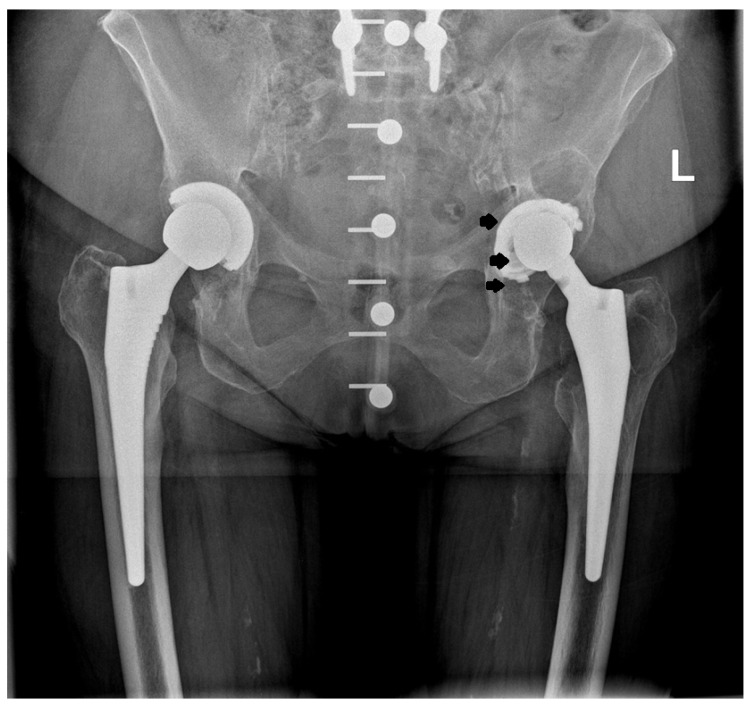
X-ray images of patient who qualified for left hip revision surgery. The arrows indicate the loosening of the acetabular element.
